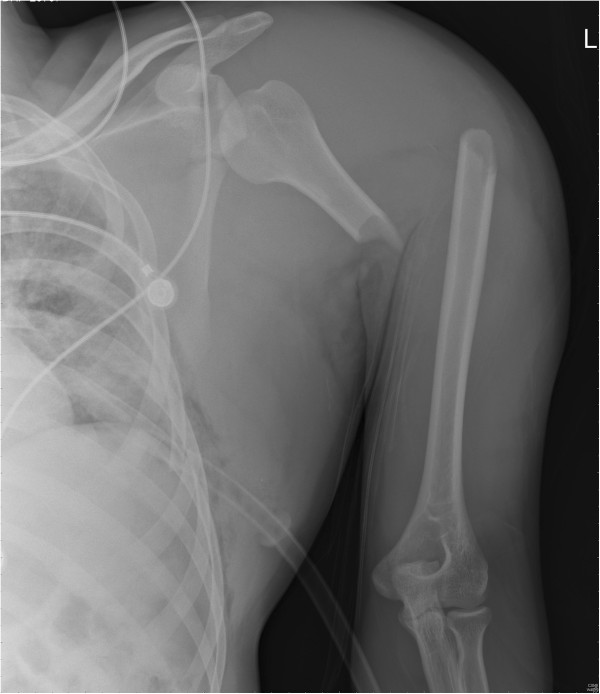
Radiograph showing fracture of humeral shaft, shoulder dislocation and scapular fracture.
